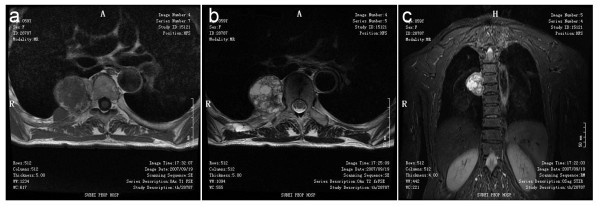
MR finding in right fifth posterior rib. MR imaging shows isointensity on a T1-weighted image (a), heterogeneous high signal pattern with a low signal rim on a T2-weighted image (b) and Coronal STIR imaging (c)
